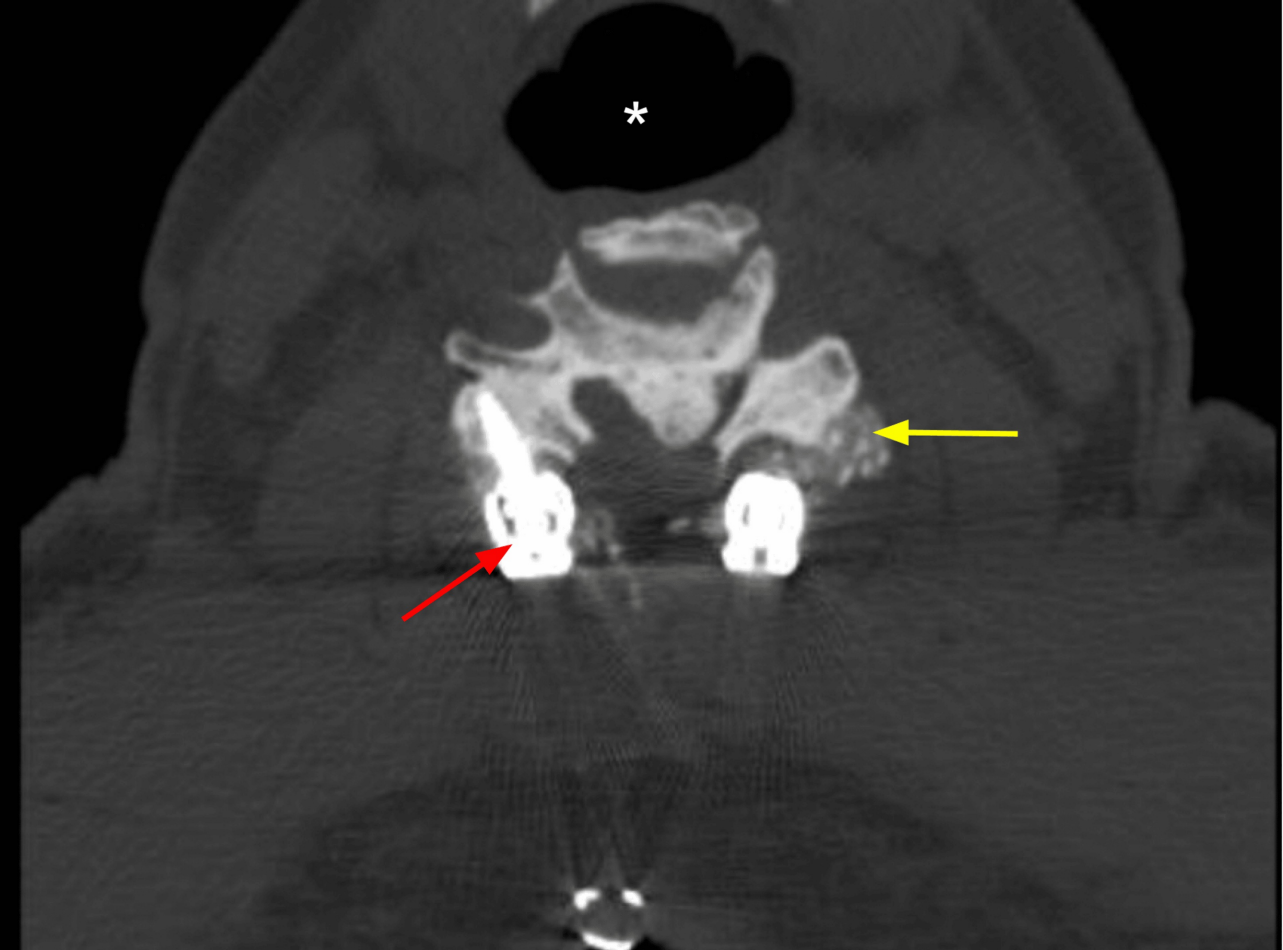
Axial view of the postoperative cervical CT scan. Imaging shows C4 vertebra with bone graft for fusion (yellow arrow) and correctly positioned screw in the lateral mass (red arrow). * trachea
